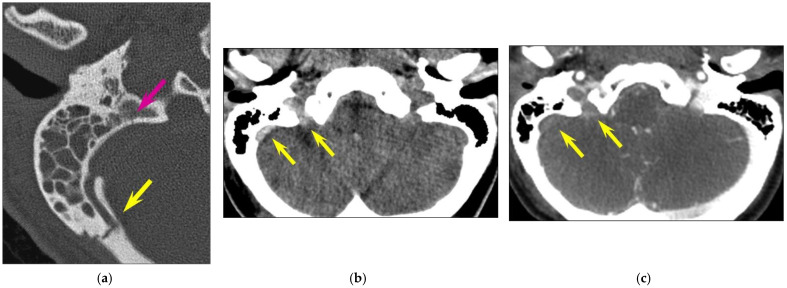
Vascular injuries. Longitudinal fracture of the right temporal bone and venous thrombosis in a 30-year-old woman involved in a motorway traffic accident. CT of the posterior fossa (a,b) unenhanced CT; (c): intravenous contrast-enhanced CT. (a) A detached fragment of the posterior part of the temporal bone, projecting into the sigmoid sinus (yellow arrow); the fracture line is also seen more medially (pink arrow). (b): spontaneous hyperdensity of the sigmoid sinus and of the jugular foramen (yellow arrows). (c) The absence of the opacification of these vascular structures, consistent with venous thrombosis (yellow arrows).
